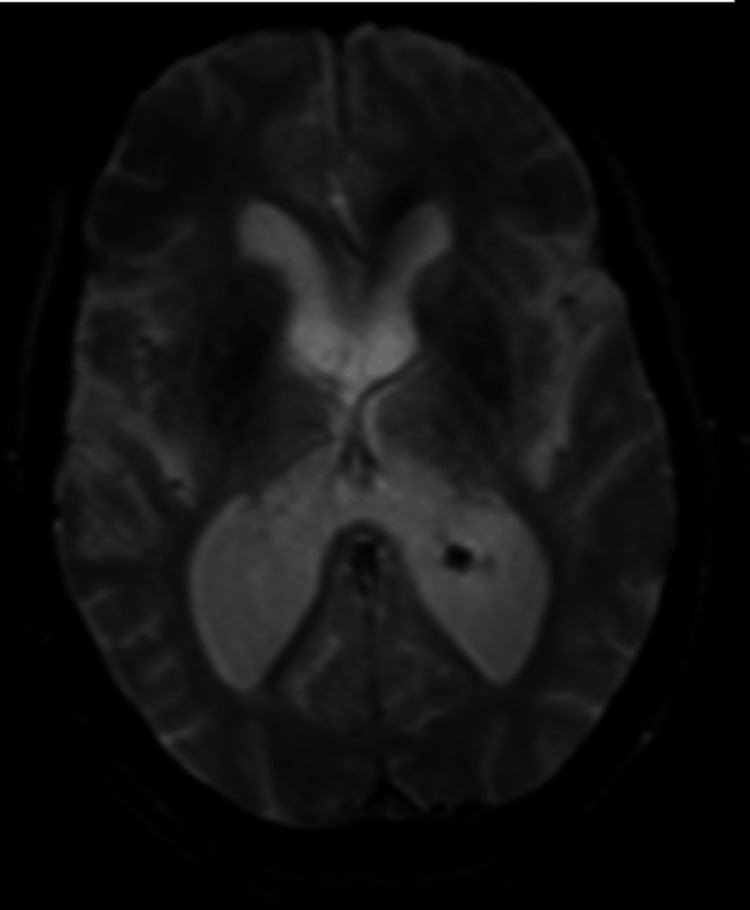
MRI An axial MRI image of the brain, obtained using a gradient echo (GRE) sequence, showing the absence of susceptibility artifacts within the ventricular and subarachnoid spaces.
